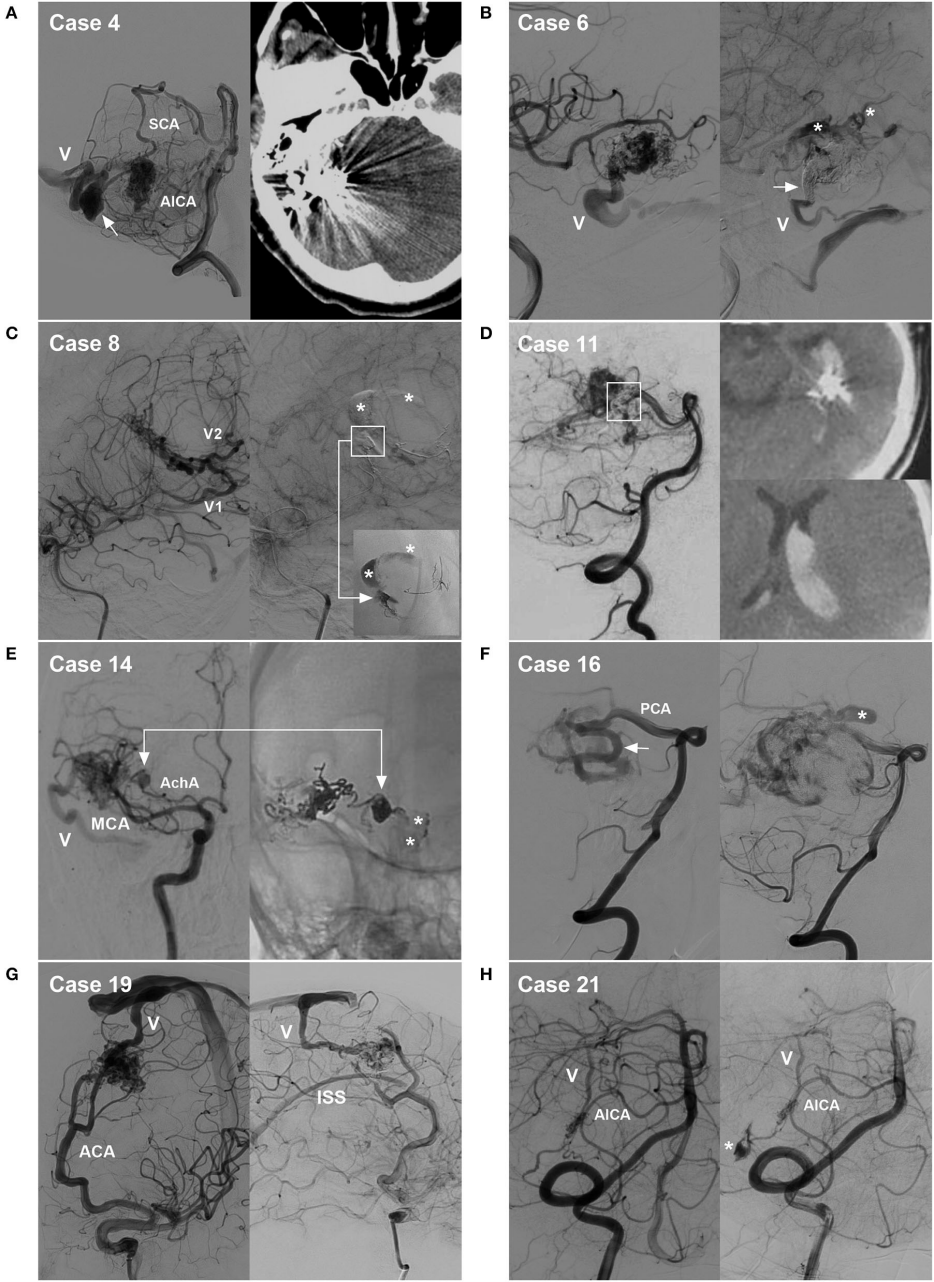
Cases of hemorrhagic complications. (A) Draining vein system rupture: left, pre-operative angiogram showing the BAVM supplied by the SCA and AICA, with the draining vein (V) and a large venous aneurysm (arrow); right, post-operative CT showing venous edema and hemorrhage. (B) Intraoperative rupture from Onyx casting: left, intraoperative angiogram showing the BAVM supplied by the MCA, with the draining vein (V) to the transverse sinus; right, angiogram of the nidus rupture showing contrast agent extravasation (asterisks) and incomplete occlusion of the draining vein (arrow). (C) Intraoperative nidus rupture from Onyx casting: left, pre-operative angiogram showing the BAVM supplied by the MCA, with two draining veins (V1 and V2); right, angiogram of the nidus rupture showing control of the hemorrhage due to continuous embolization. Ruptured point of the nidus (frame); Onyx casting (right-angled arrow, inset); and contrast agent extravasation (asterisks). (D) Post-operative hemorrhage from normal perfusion pressure breakthrough: left, post-operative angiogram showing partial nidus embolization (frame); right, post-operative CT showing intraventricular hemorrhage. (E) Intraoperative feeding artery aneurysm rupture from Onyx casting: left, pre-operative angiogram showing the BAVM supplied by the MCA and AchA, as well as an aneurysm on the AchA and the draining vein (V); right, angiogram of the aneurysm rupture showing contrast agent extravasation from the aneurysm (arrow, asterisks). (F) Non-embolized intranidal aneurysm: left, pre-operative angiogram showing remodeling and dilation of the PCA as the feeding artery (arrow); right, post-operative angiogram showing delayed rupture of the intranidal aneurysm (asterisk) left after embolization. (G) Hidden draining vein: left, pre-operative angiogram showing the BAVM supplied by the ACA and draining into the sinus (V); right, post-operative angiogram showing the hidden inferior superior sinus appearing as the draining vein after embolization. (H) Arterial perforating complication: left, pre-operative angiogram showing the BAVM supplied by the AICA, with the draining vein (V); right, intraoperative angiogram showing perforation of the feeding artery by the microguidewire (asterisk). ACA, anterior cerebral artery; AchA, anterior choroidal artery; AICA, anterior inferior cerebellar artery; BAVM, brain arteriovenous malformation; CT, computed tomography; MCA, middle cerebral artery; PCA, posterior cerebral artery; SCA, superior cerebellar artery.
